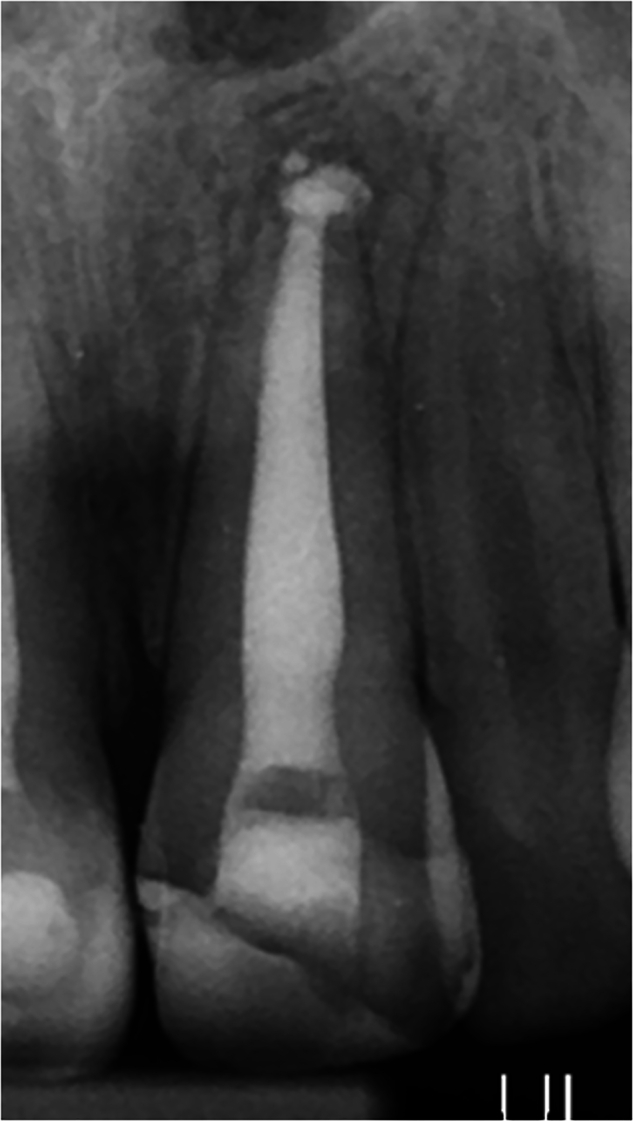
Periapical radiograph of previously treated maxillary incisor with small areas of TotalFill extrusion surrounded by ‘normal PDL’.
